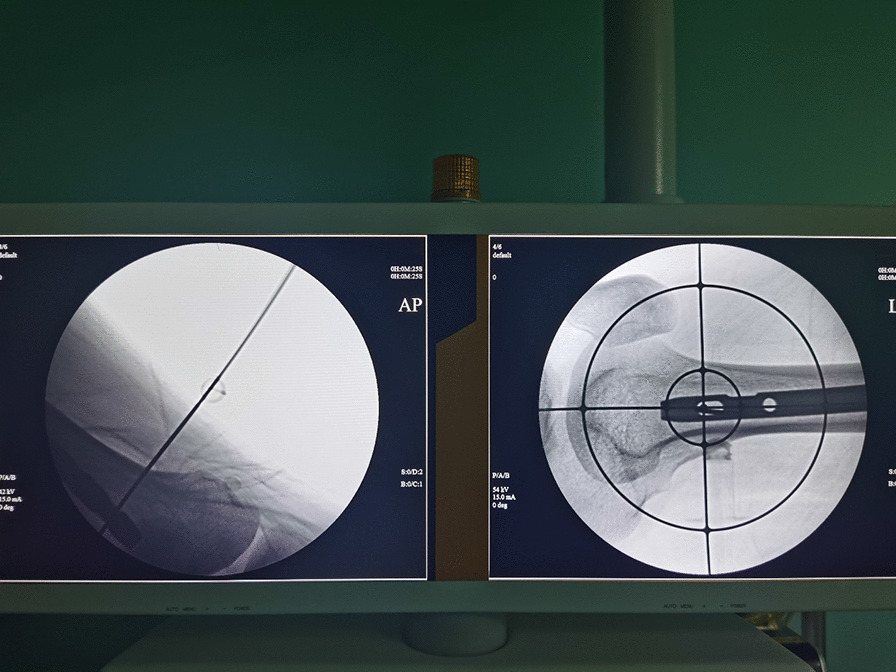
X-ray fluoroscopy confirmed that the Kirschner wire passed through the nail hole
